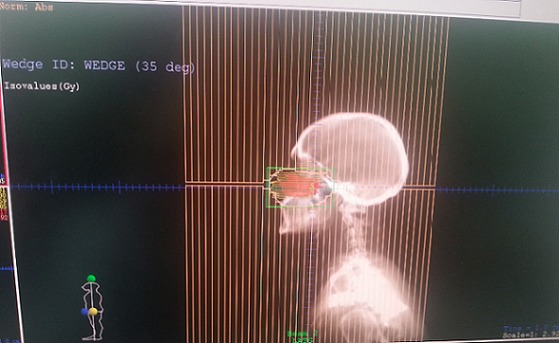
DRR (Digitally Reconstructed Radiographs) des champs d'irradiation utilisés: deux faisceaux latéraux (cas n 2)
